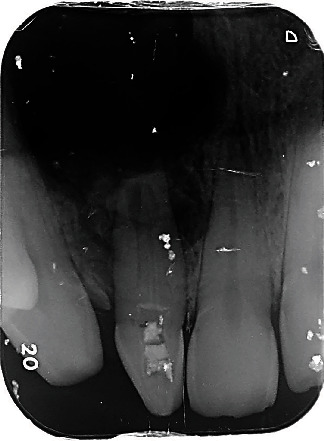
Preoperative radiograph showing a complex root canal configuration corresponding to that of type II DI associated with a large extending periapical image in relation to tooth #12.
